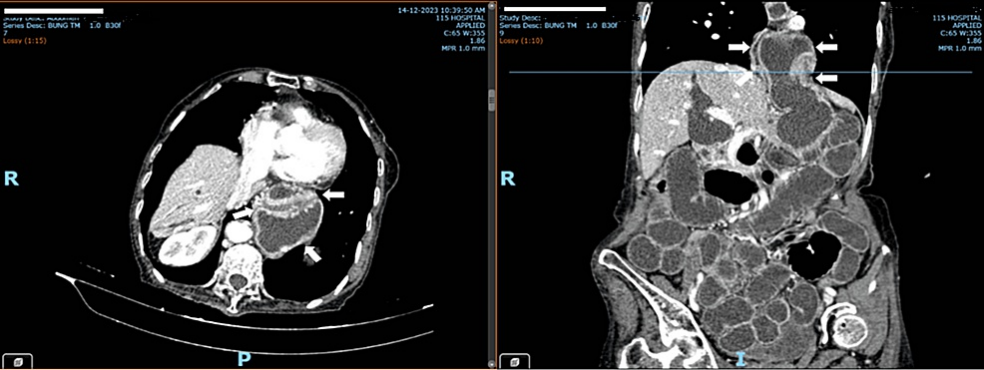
Images of the paraesophageal hernia CT scan of the axial and coronal planes after intravenous contrast injection detected gastric herniation through the esophageal opening (the arrows).
